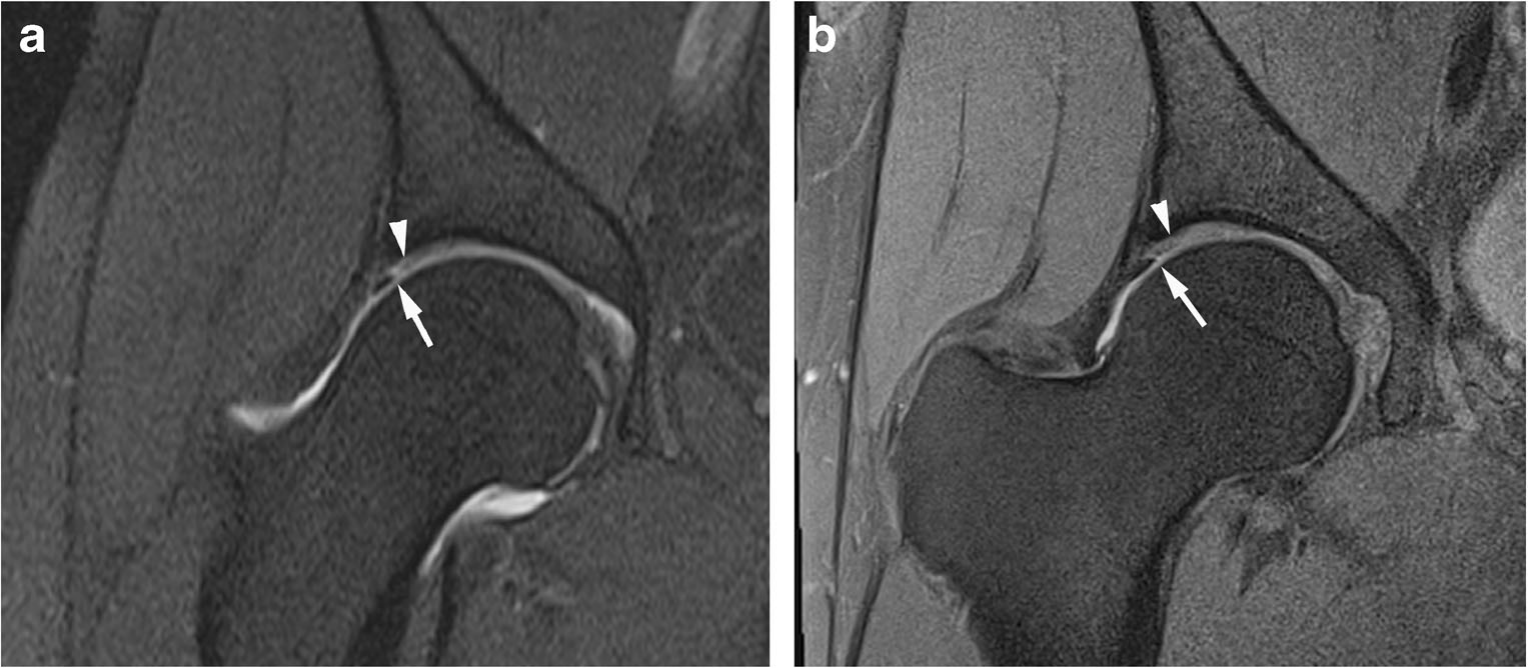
Anterosuperior acetabular labrum partial tear with adjacent cartilage delamination in a 28-year-old confirmed at surgery. Coronal (a) T1 FS 1.5T MRA image shows partial labral tear (arrow) and the adjacent cartilage shows increased linear basal fluid signal (arrowhead). (b) PD FS 3T MR image shows partial labral tear (arrow) and the adjacent cartilage shows low chondral signal (arrowhead) indicating delamination
